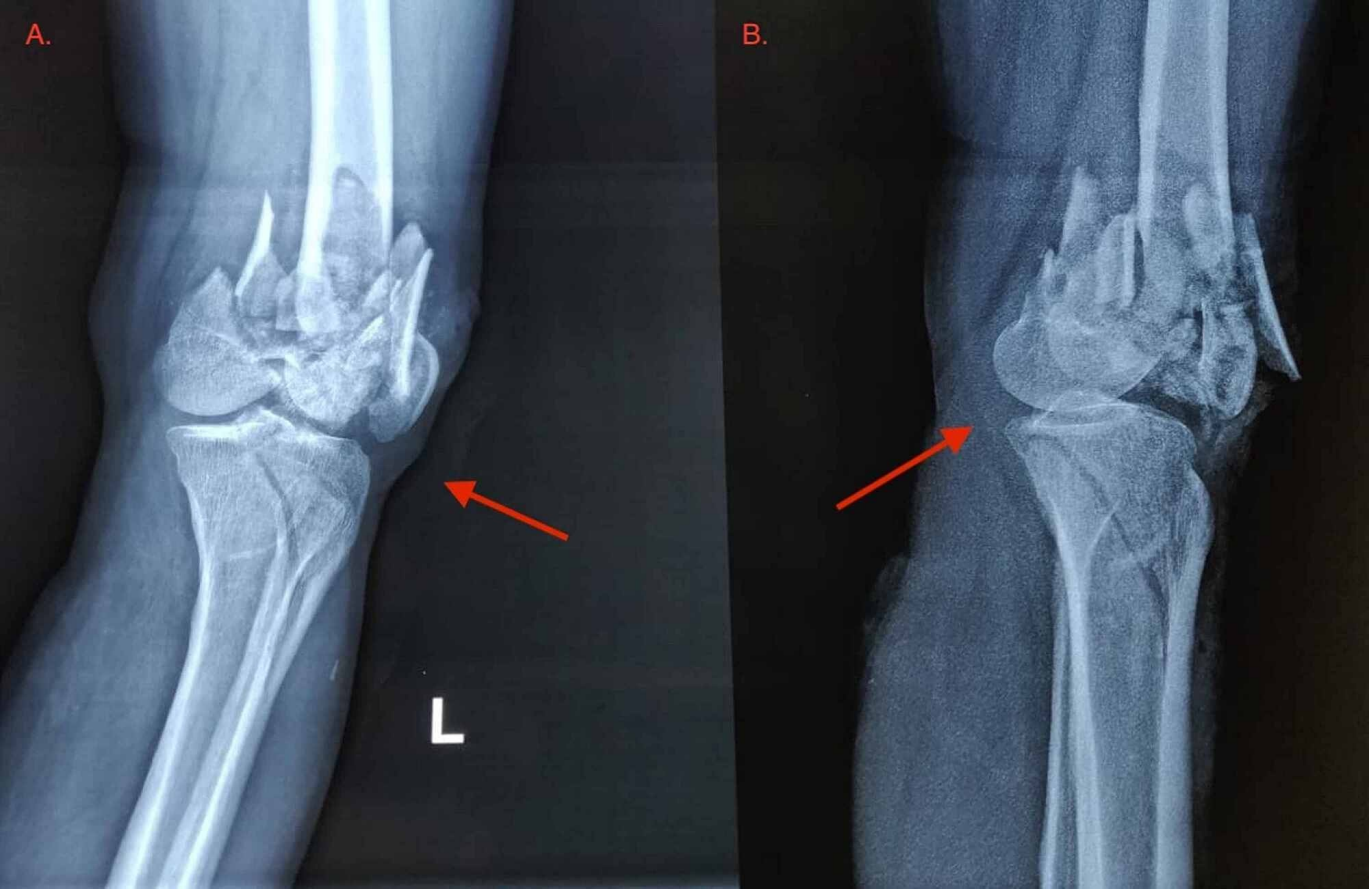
X-ray of the left knee with the leg. (A) Anteroposterior and (B) lateral views showing comminuted intercondylar fracture femur, fracture patella, and fracture proximal tibia (red arrows).
